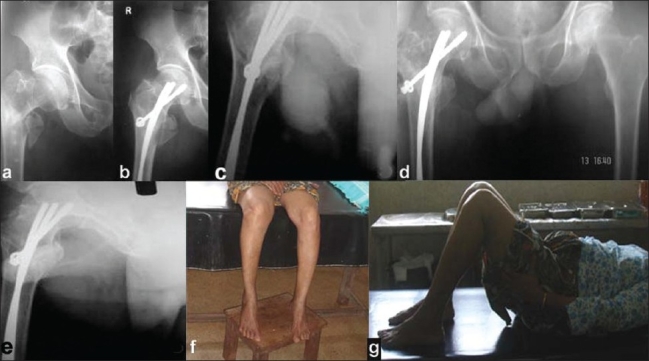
(a) Pre-operative X-ray of right hip joint of a 74-year-old female with unstable intertrochanteric fracture six weeks. Post-operative antero-posterior (b) and lateral (c) radiographs of right hip showing satisfactorily maintained fracture reduction and implant in situ. Ten months follow-up antero-posterior (d) and lateral (e) radiographs of right hip showing fracture union with good alignment and clinical photographs (f, g) showing knee and hip range of motion.
